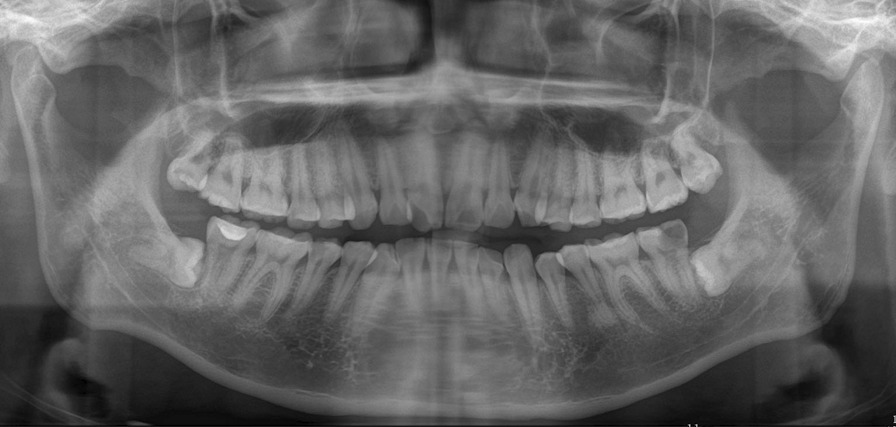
Preoperative panoramic radiographs
